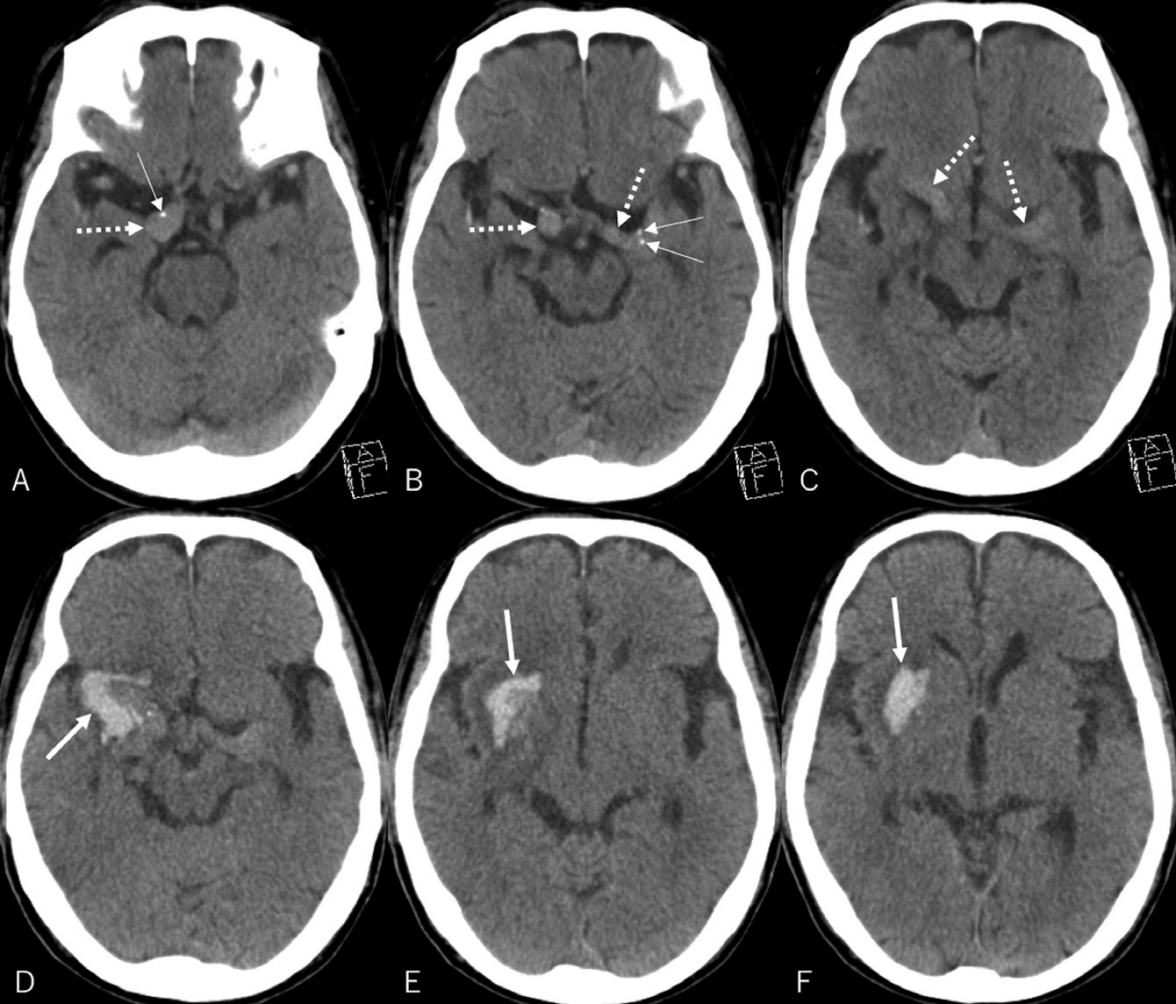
(*A-C*) Non-contrast axial computed tomography taken at presentation showing elongated masses lying in the bilateral sylvian fissures (*dotted arrows*), accompanying punctate calcifications (*thin arrows*), without hemorrhagic changes. (*D-F*) Non-contrast axial computed tomography taken 2 days later showing an irregularly shaped intraparenchymal hemorrhage, 30 × 18 × 35 mm in maximal dimension, located mainly in the right putaminal region (*arrow*).
